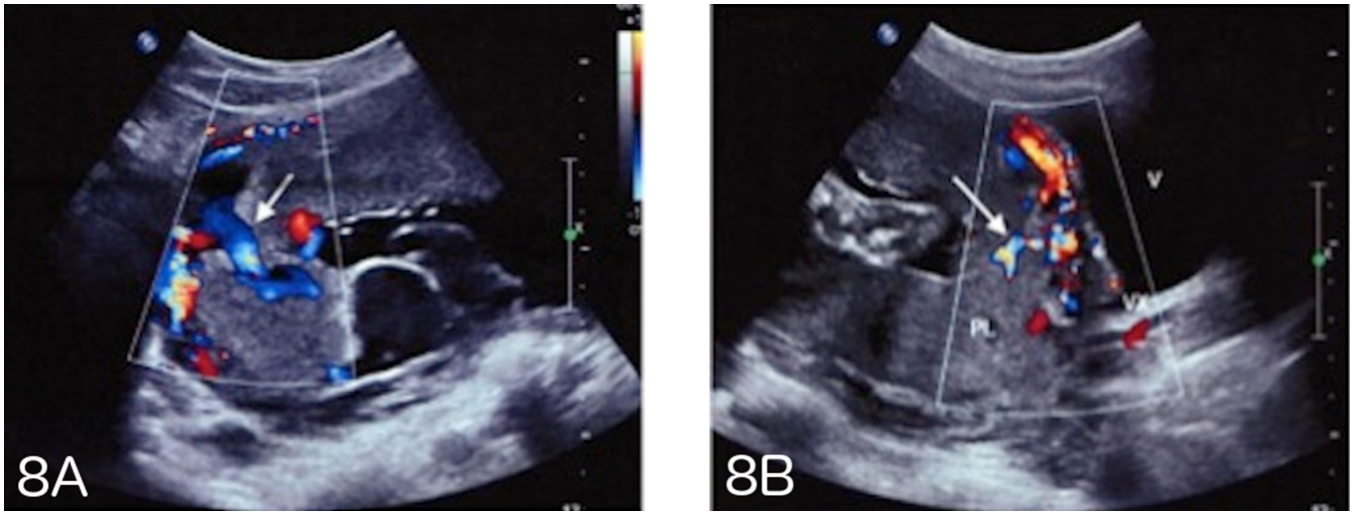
A–B - Intraplacental vascularization perpendicular to the myometrium and hypervascularization on ultrasound.
